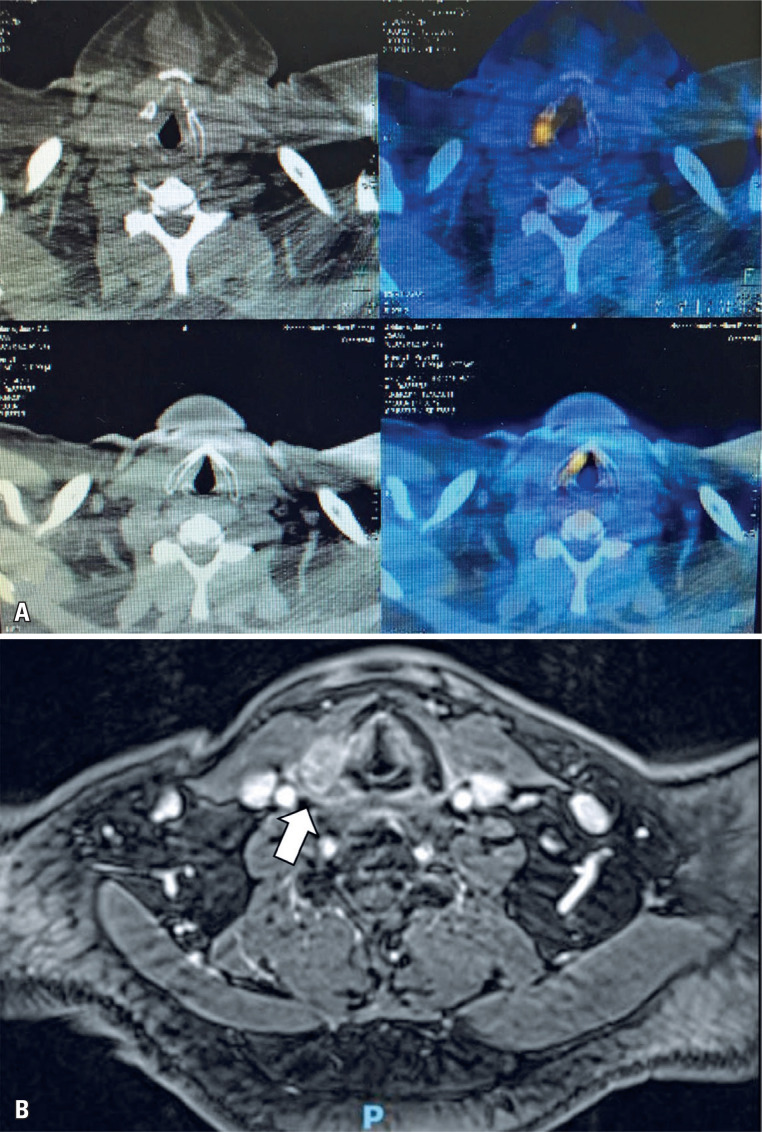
A) PET-CT revealed increased fluorodeoxyglucose (FDG) uptake in a hypermetabolic lesion involving the right thyroid cartilage ala; B) MRI showed an infiltrative lesion of the right thyroid cartilage, without invasion of other structures (arrow)
